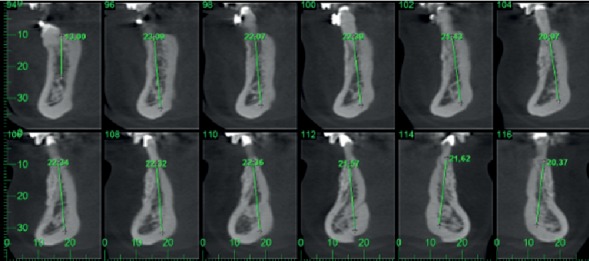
Postoperatory tomography radiograph 12 months after the horizontal bone augmentation, showing new bone formation, allowing the correct three-dimensional placement of dental implants.
